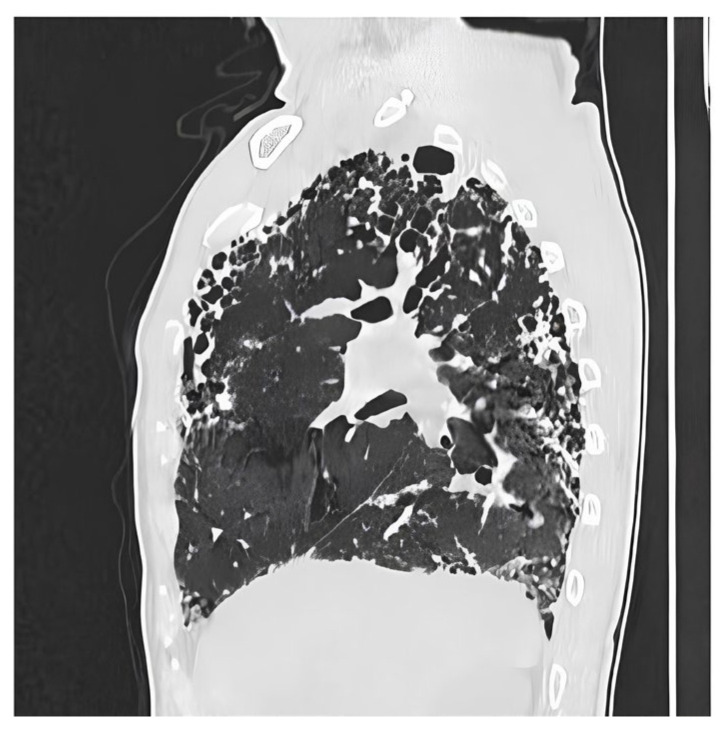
Sagittal section of a chest CT showing a scan appearance of indeterminate for Usual Interstitial Pneumonia (UIP). This sagittal HRCT image displays features of advanced pulmonary fibrosis, including prominent reticular opacities, architectural distortion, and clear evidence of honeycombing, visible as multi-layered, clustered cystic airspaces. However, the distribution of these changes is atypical for a classic Usual Interstitial Pneumonia (UIP) pattern. Instead of a distinct basal and subpleural predominance, the fibrotic abnormalities, including the honeycombing, are distributed more uniformly from the upper to the lower parts of the lung.
